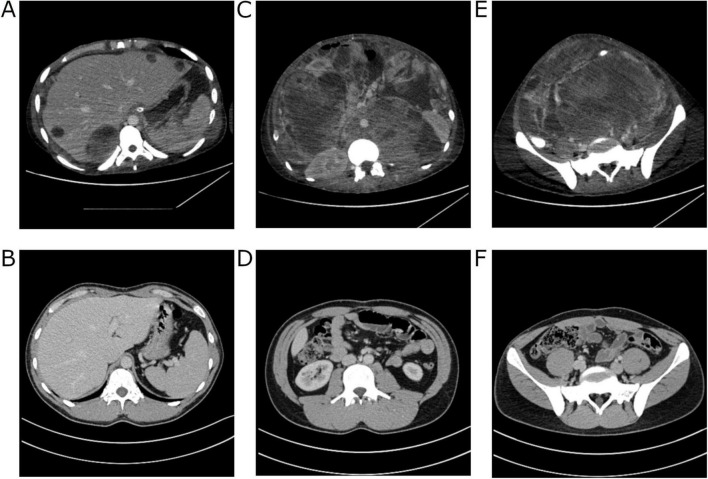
Computed tomography images of the patient’s abdomen performed at The Maria Sklodowska-Curie National Research Institute of Oncology in Warsaw. Comparison of the scan from 31.12.19 with the scan from 08.04.24. Regression of changes in the liver and peritoneal cavity (A,B). Cross-section at the level of the mid-abdomen (C,D) showing regression of the primary lesion and notable muscle tissue regeneration. Regression of changes in the pelvis (E,F).
